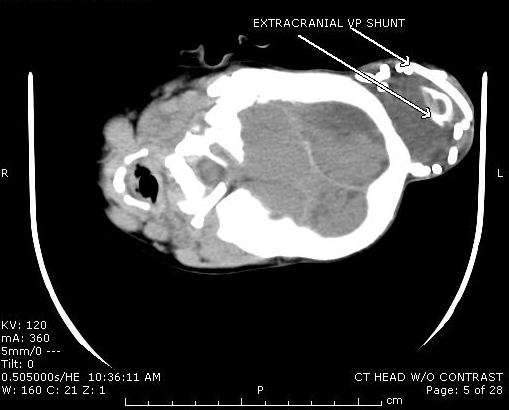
Cross Section CT Showing Coiled Shunt.
